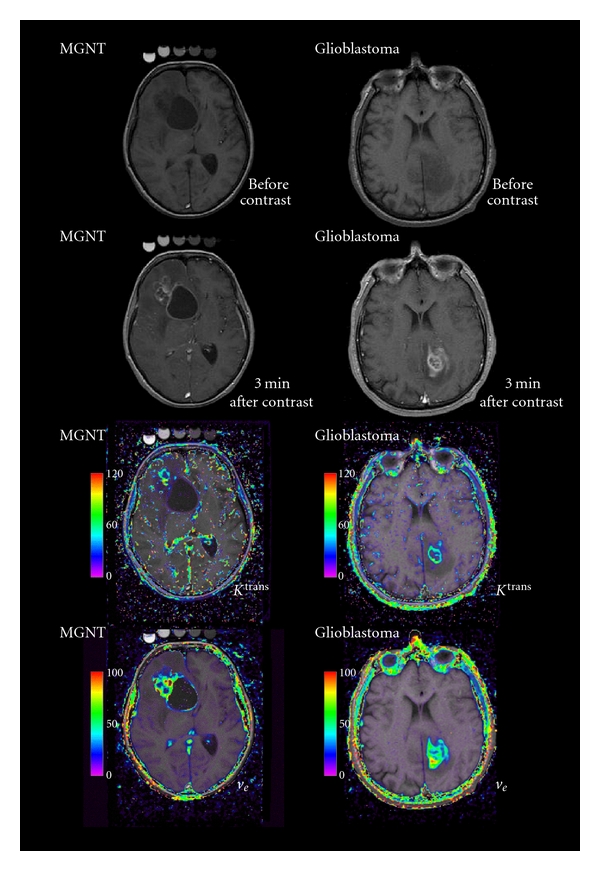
From top to bottom T1-weighted MR image before and 3 min after contrast agent injection, calculated map of transfer constant (*K*
